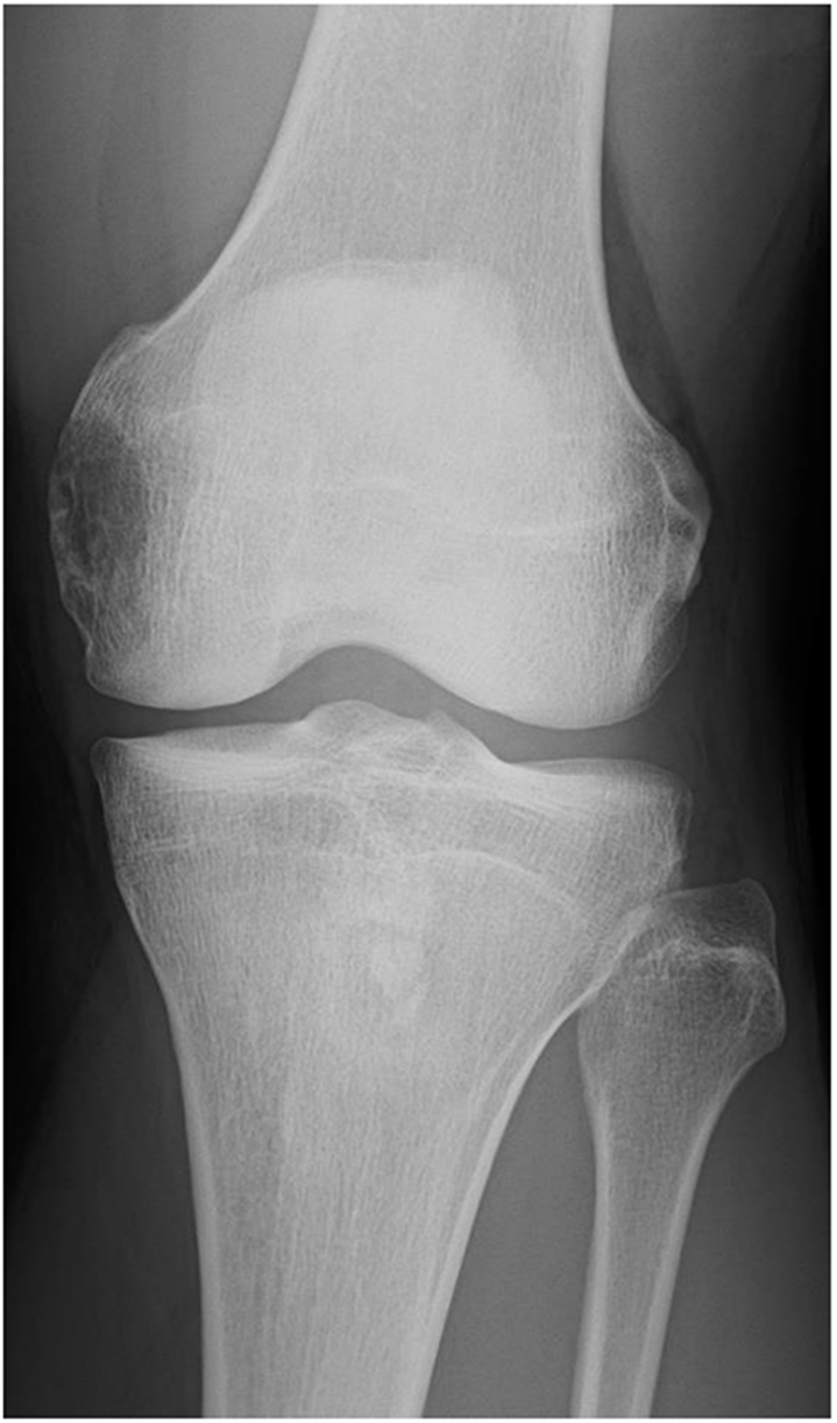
Radiograph shows osteolytic lesion in the left distal femur
